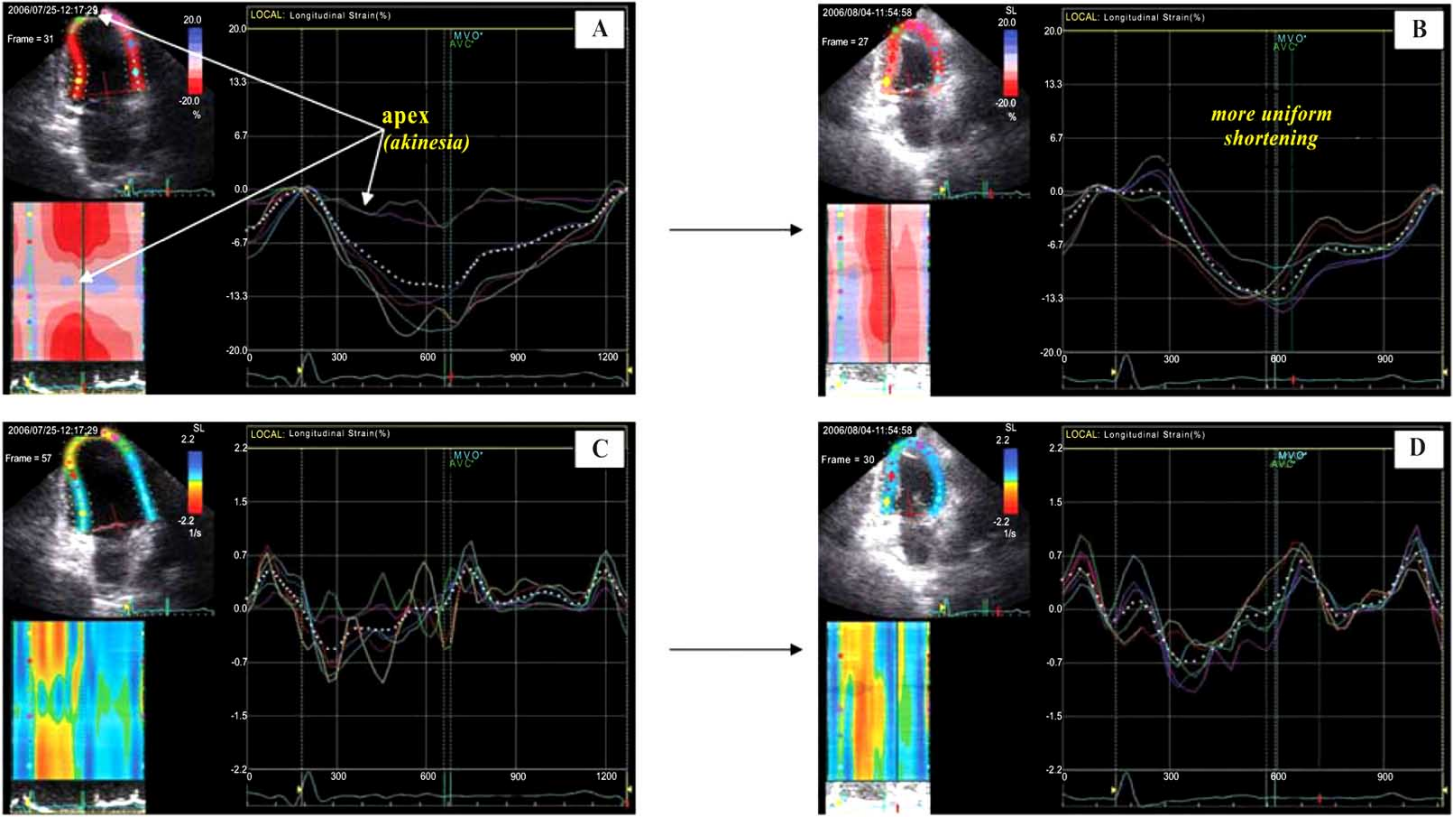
Left ventricular longitudinal strain (shortening) and strain rate (velocity of shortening) images obtained from apical 2-chamber views before (A and C, respectively) and after surgical ventricular restoration (B and D, respectively) in a patient with initially severe LV dysfunction after apical myocardial infarction. Panels B and D show more uniform shortening (amplitude and velocity, respectively) after surgery. [Knosalla C, Dandel M *et al.*, Annual Meeting of the German Society for Thoracic and Vascular Surgery 2007].
